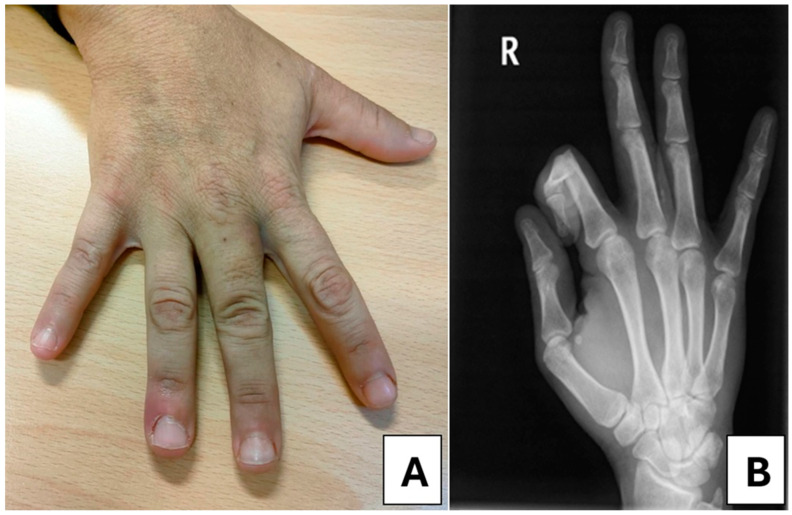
(A) Photograph of the right hand demonstrating the resolution of the calcified mass after treatment; (B) X-ray of the right hand showing the absence of calcifications and the full resolution of the condition.
